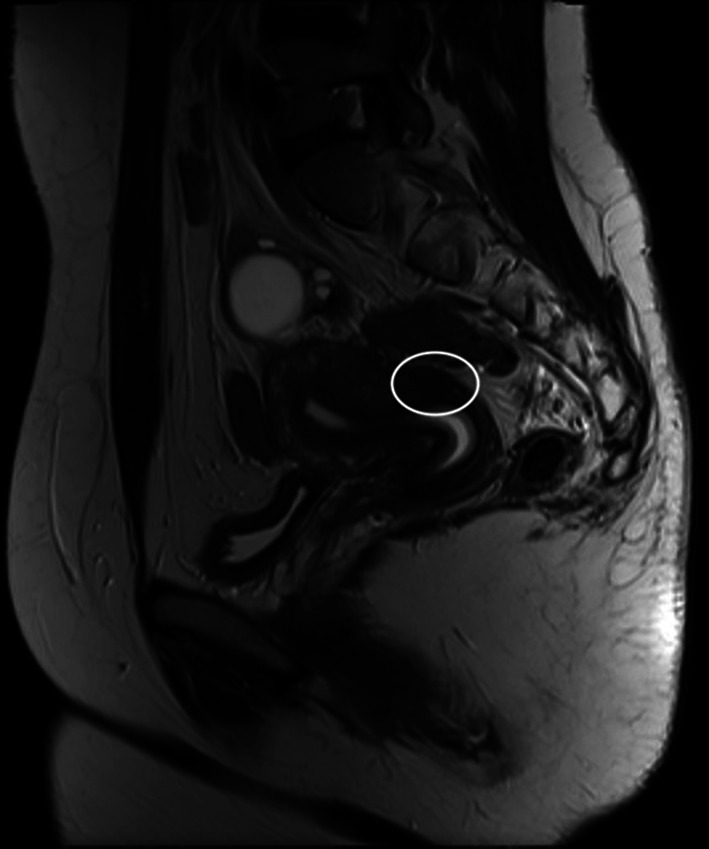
T2 weighted sagittal MRI image showed involvement of retrocervical region as a site of DIE with some adhesions in the posterior cul-de-sac
